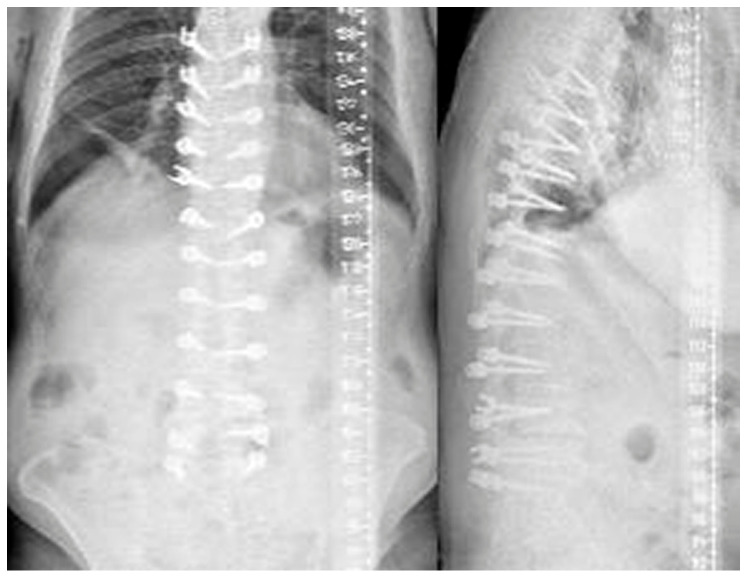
Pre- and postoperative AP and lateral radiographs showing multilevel posterior stabilization and alignment restoration.
